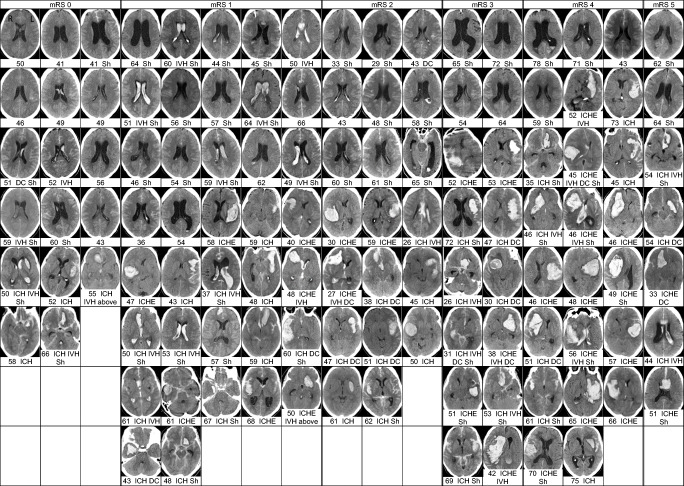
Primary CT scans of the 120 aSAH patients alive at 3 years, arranged according to their mRS. A total of 269 aSAH patients had been acutely admitted in poor condition (H&H 4; *n*=145 or H&H 5; *n*=124) to the neurointensive care between 2005 and 2015 from Eastern Finnish catchment population (Fig. 1). At 3 years, 120 (45%) of them were alive. This presents one representative CT slice of each patient (age in years) on admission, arranged according to the patient’s mRS from 0 to 5 at 3 years. There were 71 (59%) intracerebral hematomas (ICHs) on admission, 29 (41%) of them microsurgically evacuated (ICHE). There were 31 (26%) intraventricular hematomas (IVHs). Extraventricular drainage (EVD) was used in 105 (88%) patients and not used in 15 (13%). A total of 16 (13%) decompressive craniectomies (DC) were performed. In two patients in poor condition (mRS 5), cranioplasty was not performed. A ventriculoperitoneal shunt (Sh) was subsequently inserted in 55 (46%) patients. The collection is zoomable to observe details in different CT slices. By using the Find command, the following can be simultaneously identified in the whole collection: mRS, ICH, ICHE, IVH, DC, or Sh, or their different combinations. aSAH, aneurysmal subarachnoid hemorrhage; H&H, Hunt and Hess scale; CT, computed tomography; mRS, modified Rankin Scale; ICH, intracerebral hematoma; ICHE, intracerebral hematoma microsurgically evacuated; IVH, intraventricular hematoma (blood clot); EVD, extraventricular drainage; DC, decompressive craniectomy; Sh, shunt. Small blood sedimentations in occipital tips of the lateral ventricles were not considered as intraventricular clots (see above IVH)
